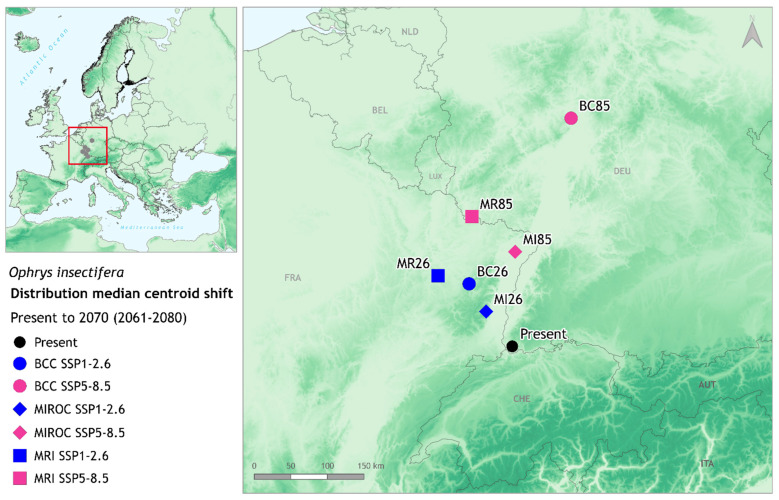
Median centroids for current and future projected distributions of *Ophrys insectifera*, for the EnvThin ensemble in 2070 time slice. Black point represents the current distribution’s median centroid, the blue points stand for the future best-case scenario (SSP1-2.6), and the magenta points for the future worst-case scenario (SSP5-8.5). GCMs differentiate by shape: BCC—circle, MIROC—diamond, MRI—square. Map is designed in QGIS v.3.18 ‘Zürich’, using ETRS89—Lambert Conformal Conic Coordinate Reference System.
